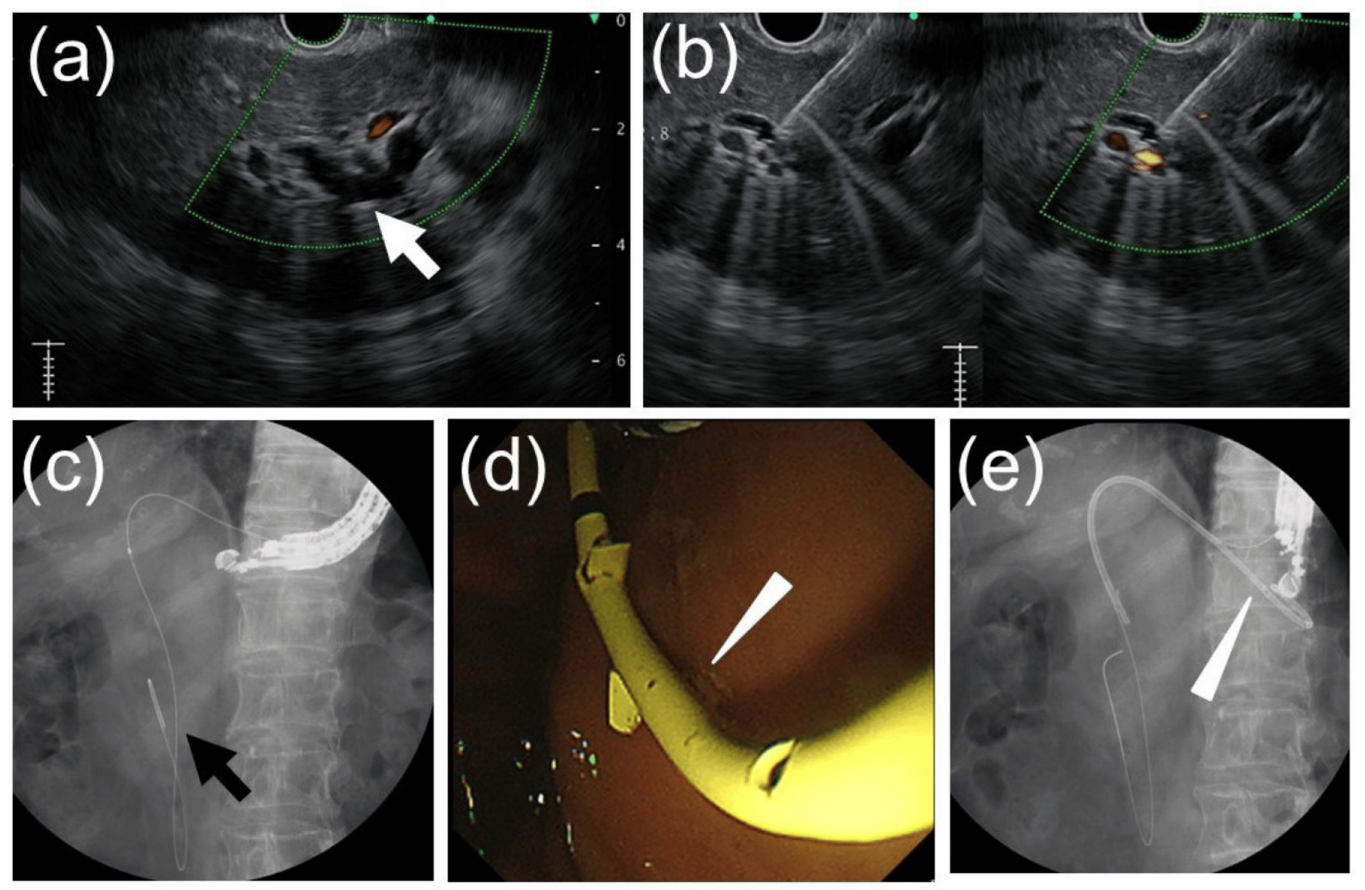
Images obtained during endoscopic ultrasound-guided hepaticogastrostomy (a) The dilated left intrahepatic bile duct (white arrow) was visualized using an ultrasound endoscope from the stomach. (b) The dilated left intrahepatic bile duct was punctured under endoscopic ultrasound guidance. (c) A guidewire (black arrow) was inserted into the bile duct and advanced beyond the duodenal papilla into the duodenal lumen. (d) An additional guidewire was advanced into the bile duct, and a plastic stent (white arrowhead) was inserted transgastrically into the bile duct, with one guidewire left in place. (e) A plastic stent (white arrowhead) was deployed from the stomach into the bile duct, successfully completing endoscopic ultrasound-guided hepaticogastrostomy.
